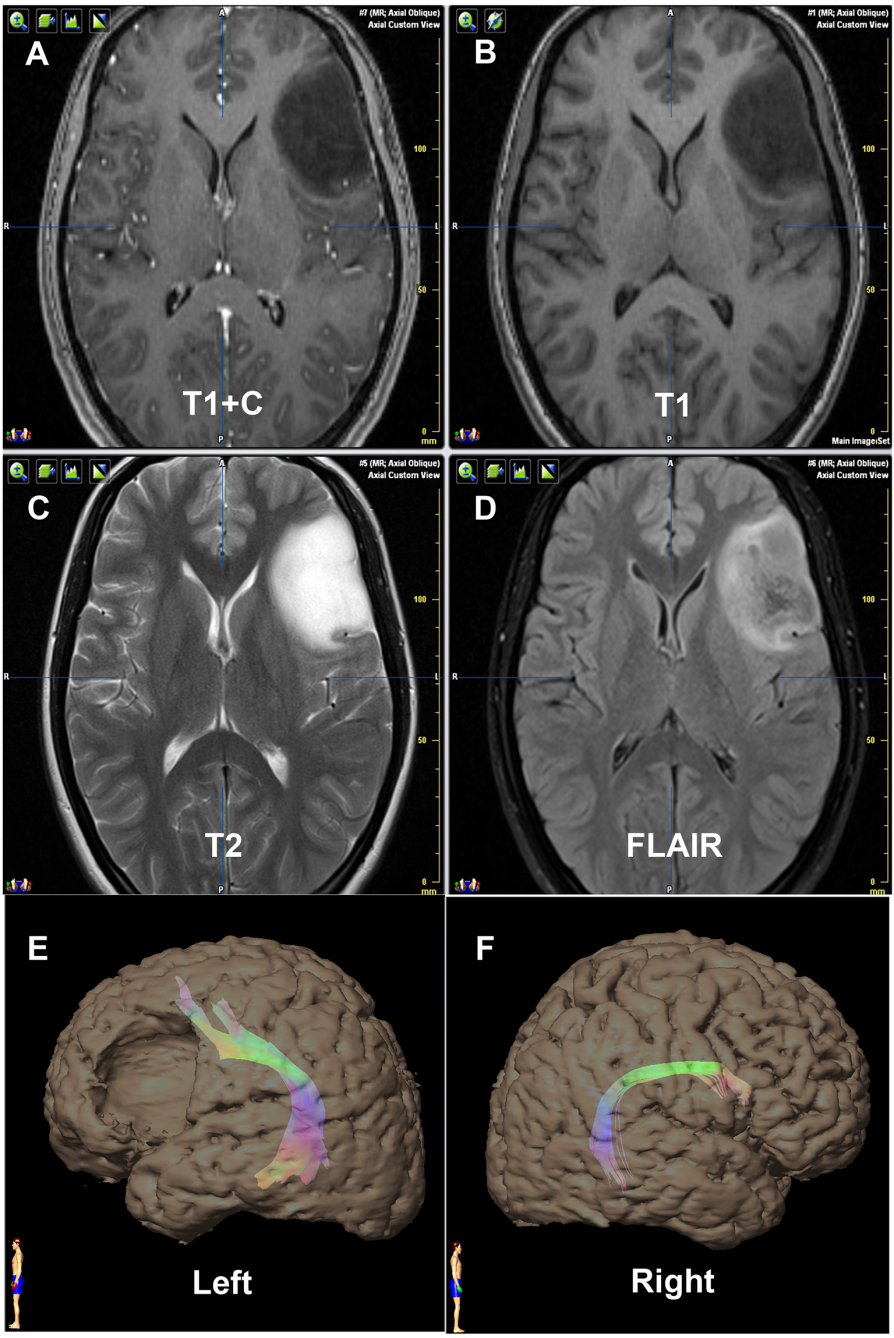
Contrast of bilateral AF by DTT of Patient No. 9. Panels (A–D) are preoperative MRI T1+C, T1, T2, and FLAIR, respectively, on one same transverse slice of Patient No. 9, who was diagnosed as primary astrocytoma in insular and temporal lobe. Panels (E, F) show that the relative more fiber number and volume of AF are on the left hemisphere, which are in concordance with the language dominant side of Wada test.
